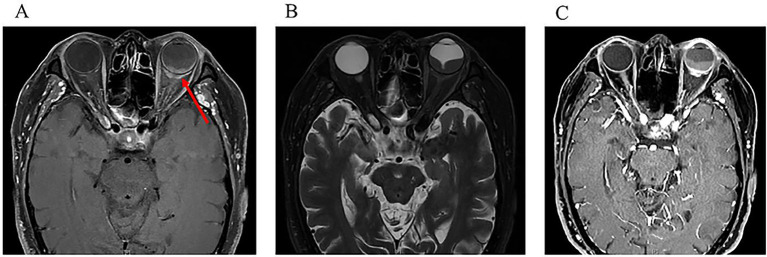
One year after initial presentation. An MRI scan of the orbits showed a mass (arrow) of irregular shape, predomi-nantly affecting the posterior parts of the eyeball, as well as transscleral extension. (A) Horizontal view of T1-weighted sequences; (B) Horizontal view of T2-weighted se-quences; (C) Horizontal view on contrast-enhanced in T1-weighted sequences.
